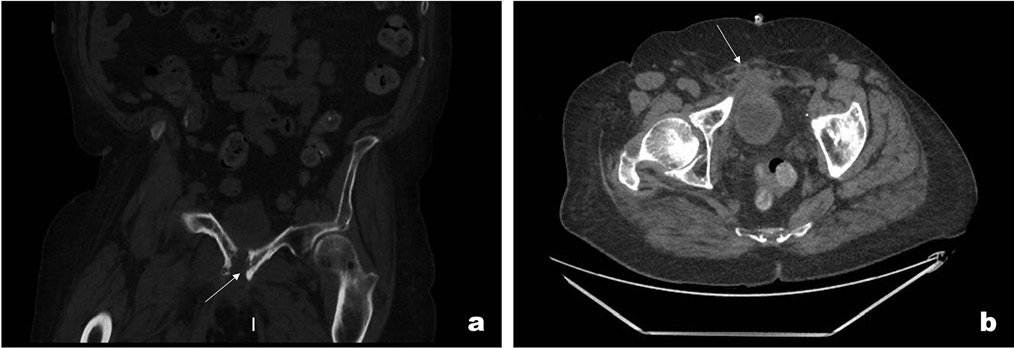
(a) Post-contrast CT coronal (bone window) slice showing cortical destruction involving the pubic bone bilaterally in keeping with osteomyelitis. (b) CT axial (soft tissue window) slices showing fluid surrounding the bladder and pubic symphysis with surrounding fat stranding.
